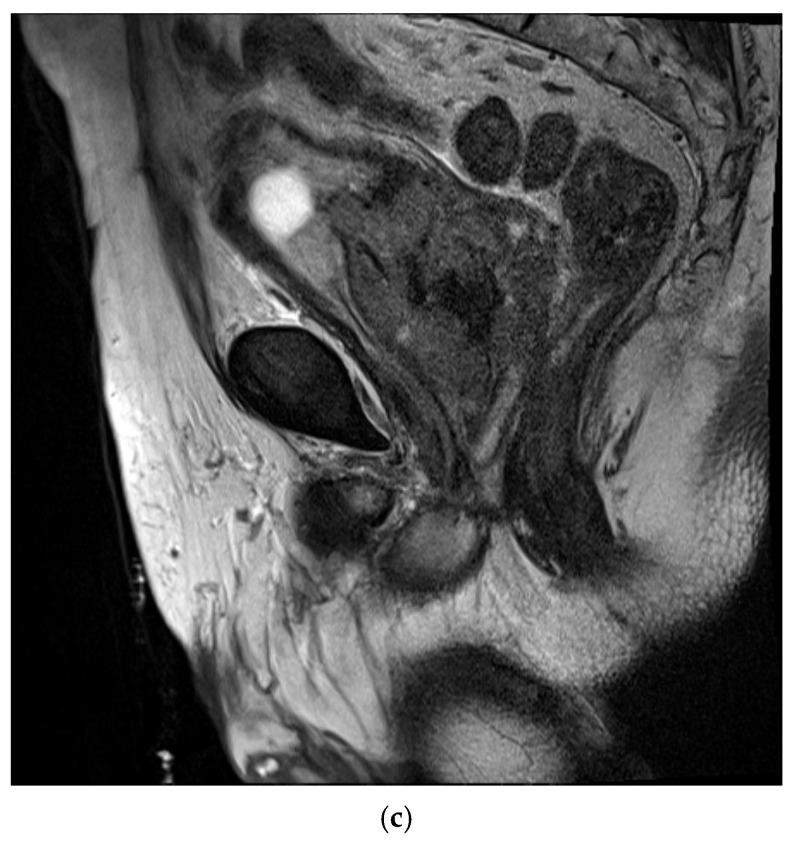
Baseline radiologic findings demonstrating locally advanced prostate squamous cell carcinoma. (a) Axial contrast-enhanced computed tomography (CT) image showing asymmetric enlargement of the prostate with heterogeneous enhancement and direct invasion of the urinary bladder. (b) Sagittal contrast-enhanced CT image showing diffuse prostatic tumor extension with bladder and seminal vesicle invasion. (c) Multiparametric magnetic resonance imaging (MRI) revealing diffuse prostate tumor infiltration with bladder and seminal vesicle invasion and close abutment to the anterior rectal wall.
